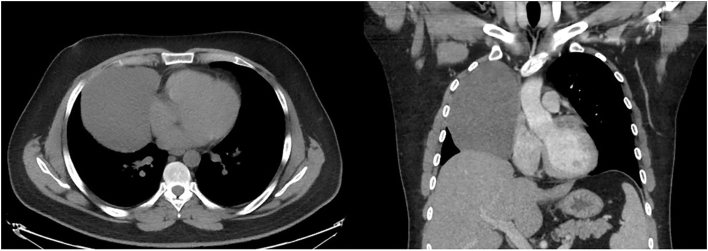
Chest computed tomography demonstrating a cystic mass in the anterior mediastinum.
